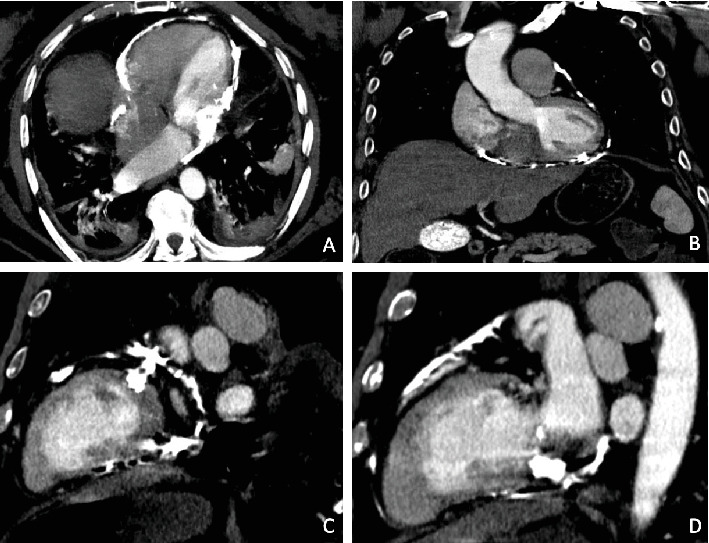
CT thorax with contrast showing pericardial calcifications. (A) Axial view, (B) coronal view, (C) sagittal view showing posterolateral aspects of the heart, and (D) sagittal view showing anteroseptal aspects of the heart.
